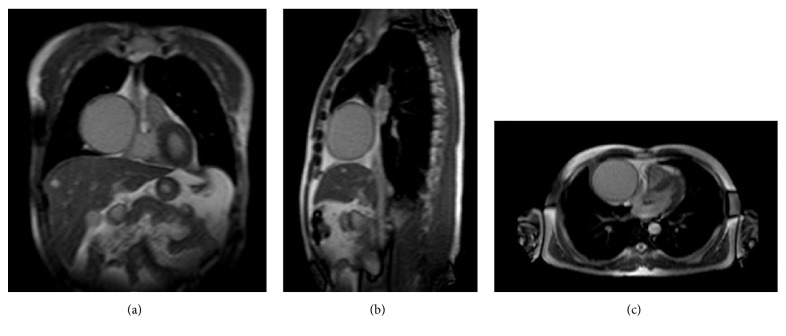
Coronal (a), sagittal (b), and transverse (c) views of thoracic MRI show a 9 cm pericardial cyst located at the right cardiophrenic angle compressing the right atrium.
